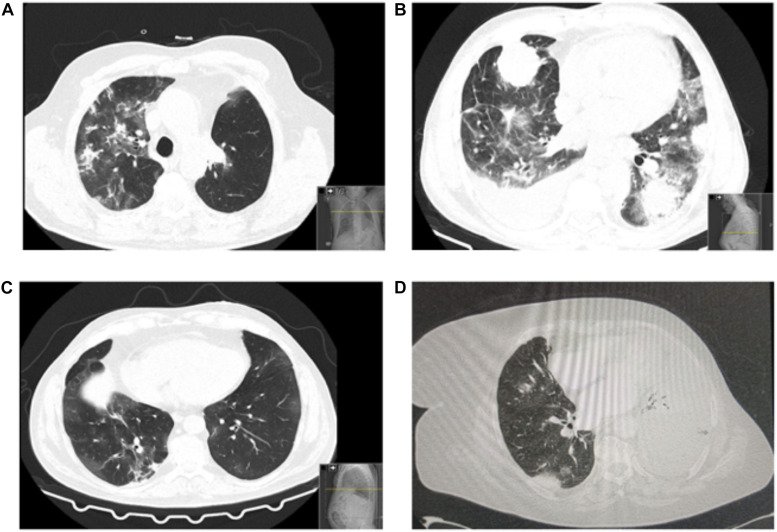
Patients’ chest CT scans. (A) Bilateral predominantly peripheral ground-glass opacities. Asymmetrical consolidations in the right lobes suggestive of concomitant bacterial infection. Left upper lobe lobectomy signs (consistent with recent surgery). (B) Patient with multiple findings related to coronavirus disease 2019 (COVID-19) and the underlying lung cancer: bilateral ground-glass opacities, pulmonary mass and pleural implant, right pleural effusion. (C) Chest CT: bilateral ground-glass opacities, both central and peripheral, some with nodular shape. Some “band” consolidative opacities in the right upper lobe, middle lobe, and lower inferior lobe opacities suggestive of residual processes. (D) Left large-volume pleural effusion with left lung atelectasis. Diffuse ground-glass opacities. Small right pleural effusion. No signs of pulmonary thromboembolism.
